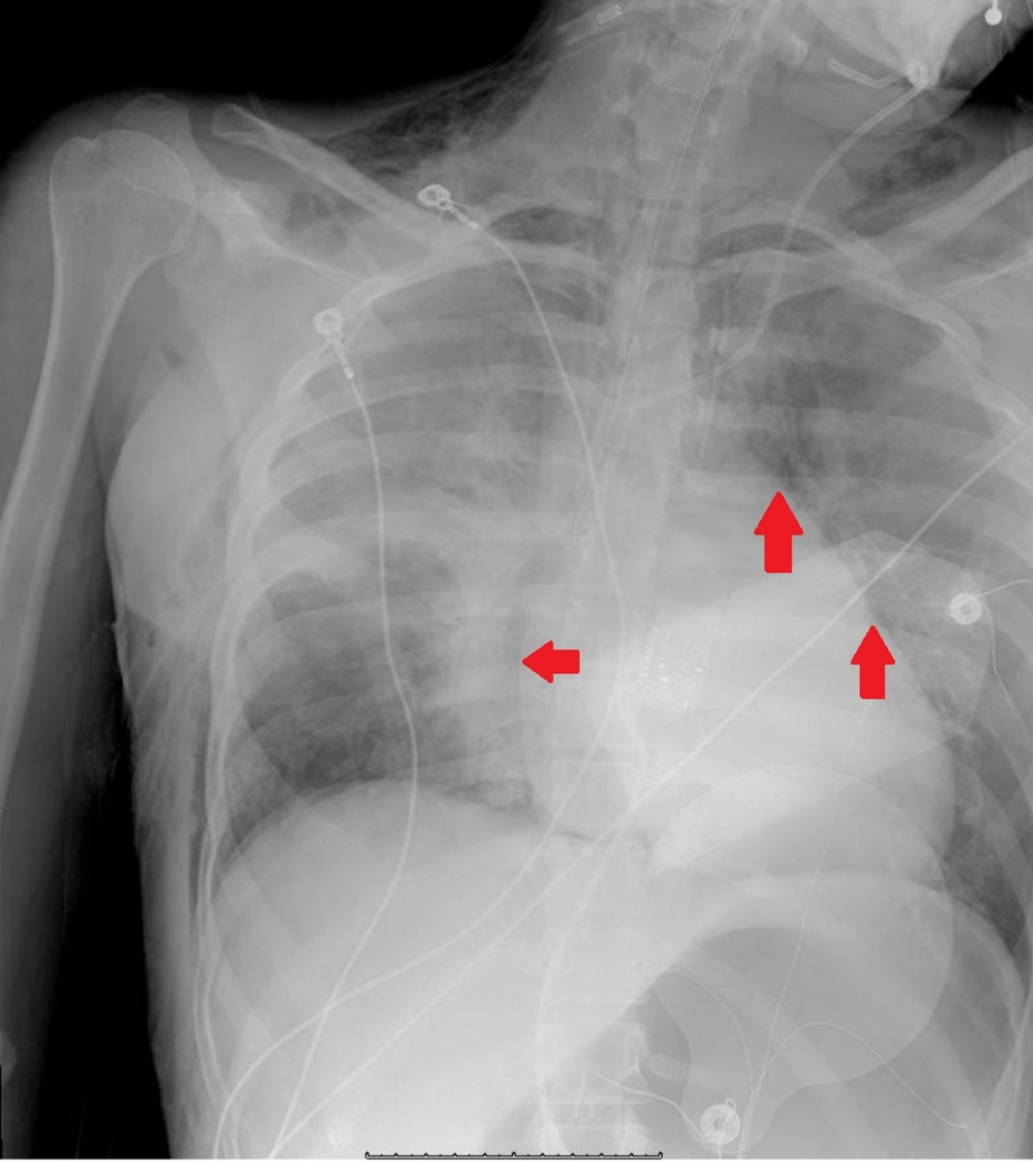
Chest X-ray showing the presence of pneumomediastinum Red arrows point to free air around the heart silhouette.
